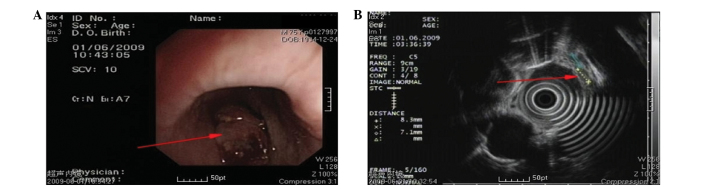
Endoscopic ultrasonography revealing (A) an esophageal neoplasm (indicated by the red arrow) and (B) multiple enlarged lymph nodes on the outside of the esophagus wall (indicated by the red arrow).
